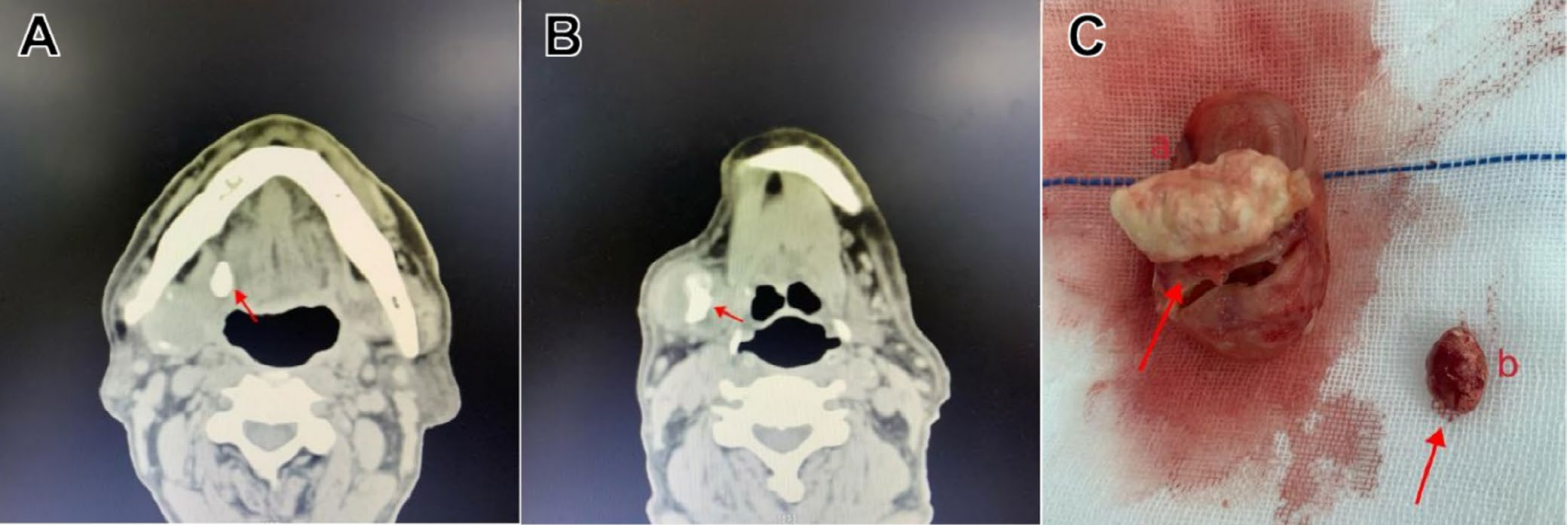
Location of the patient's lithiasis. Preoperative maxillofacial CT examination: (A) Lithiasis in the submandibular gland duct; (B) Lithiasis in the submandibular gland; (C) Lithiasis removed from the submandibular gland and lithiasis removed from the submandibular gland duct. The red arrow indicates the location of the lithiasis.
